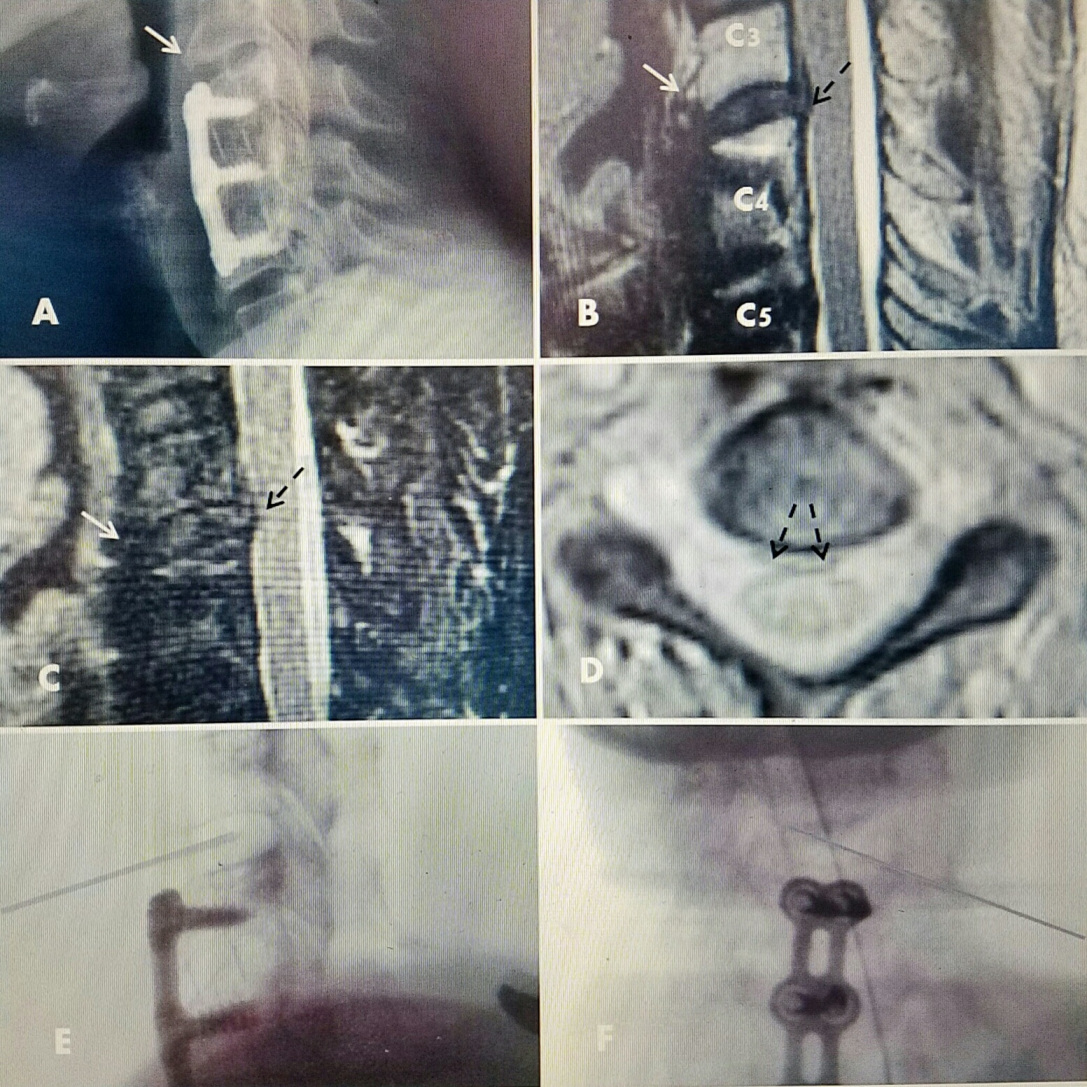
Patient #1 with C3-4 herniated disc above previous C 4-C 6 fusion A: Plain X-rays showing solid C4-C6 ACDF with screws and plate with interbody fusion performed five years previously. Normal C3-4 alignment and disc height. Beginning anterior spur C3 (white arrow). B: T2 MRI showing C3-4 herniated disc (dotted black arrow) without endplate change or narrowing but beginning ventral spur anteriorly C3 (white arrow). C: MRI Sagittal STIR image: clear C3-4 disc herniation (dotted black arrow) with small superior extension behind C3 vertebral body. D: Axial T2 MRI, small C3-4 disc herniation midline and slightly to right of midline (dotted black arrows). There are no bone or degenerative changes in the foramina or facet joints and no foraminal narrowing. E/F: 1.3 mm discectomy probe in C3-4 disc space. ACDF: Anterior cervical discectomy and fusion; MRI: Magnetic resonance imaging.
